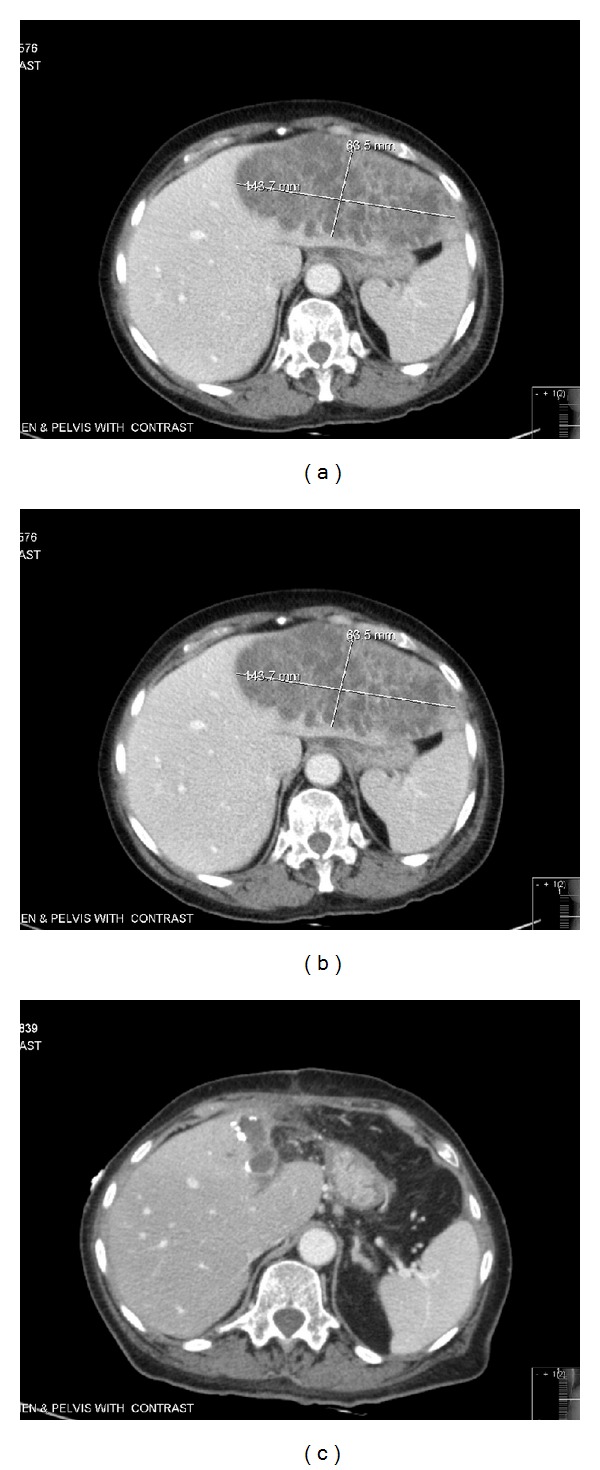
(a) Computed tomographic scan of the abdomen pelvis prior to IR drainage showing left liver abscess measuring 13.5 × 7.4 × 10.3 cm. (b) Computed tomographic scan of the abdomen pelvis 2 days after the IR drainage of 200 cc fluid, showing increase in abscess size to 16 × 7 cm. (c) Computed tomographic scan of the abdomen pelvis 5 weeks following left partial liver lobectomy and antibiotics showing abscess size reduced to 5.3 × 3.9 × 7 cm.
